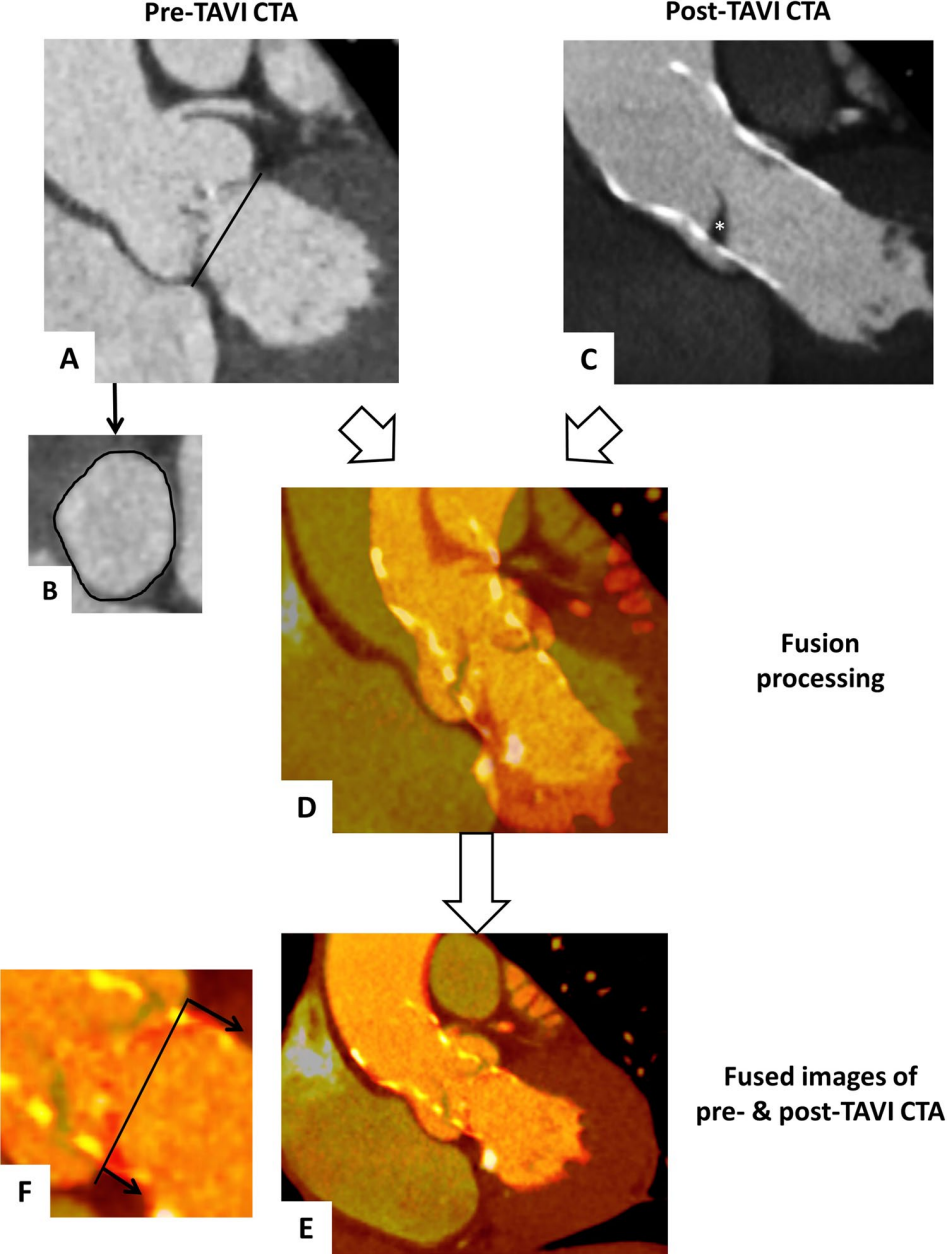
Visualization of fusion imaging process. Pre-TAVI CTA sagittal oblique (a) and axial (b) reconstructions with delineated annulus plane and post-TAVI CTA sagittal oblique reconstruction with the implanted Evolut R (c, * marked a leaflet thrombosis*)*. After semi-automatically merging of the pre- and post-TAVI CTAs (d), we manually adapted the fused images for an optimal alignment of the device-landing zone (e). Finally, the THV distances below the native annulus were measured next to all three cusps to assess the prosthesis implantation depth (f, *arrows for distance measurements)*.—marked the annulus plane
